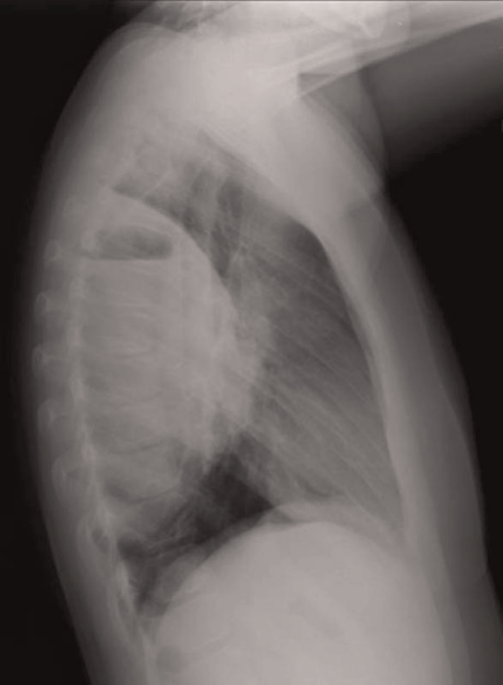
Lateral chest X-ray revealed opacification along superior margin of cyst.
